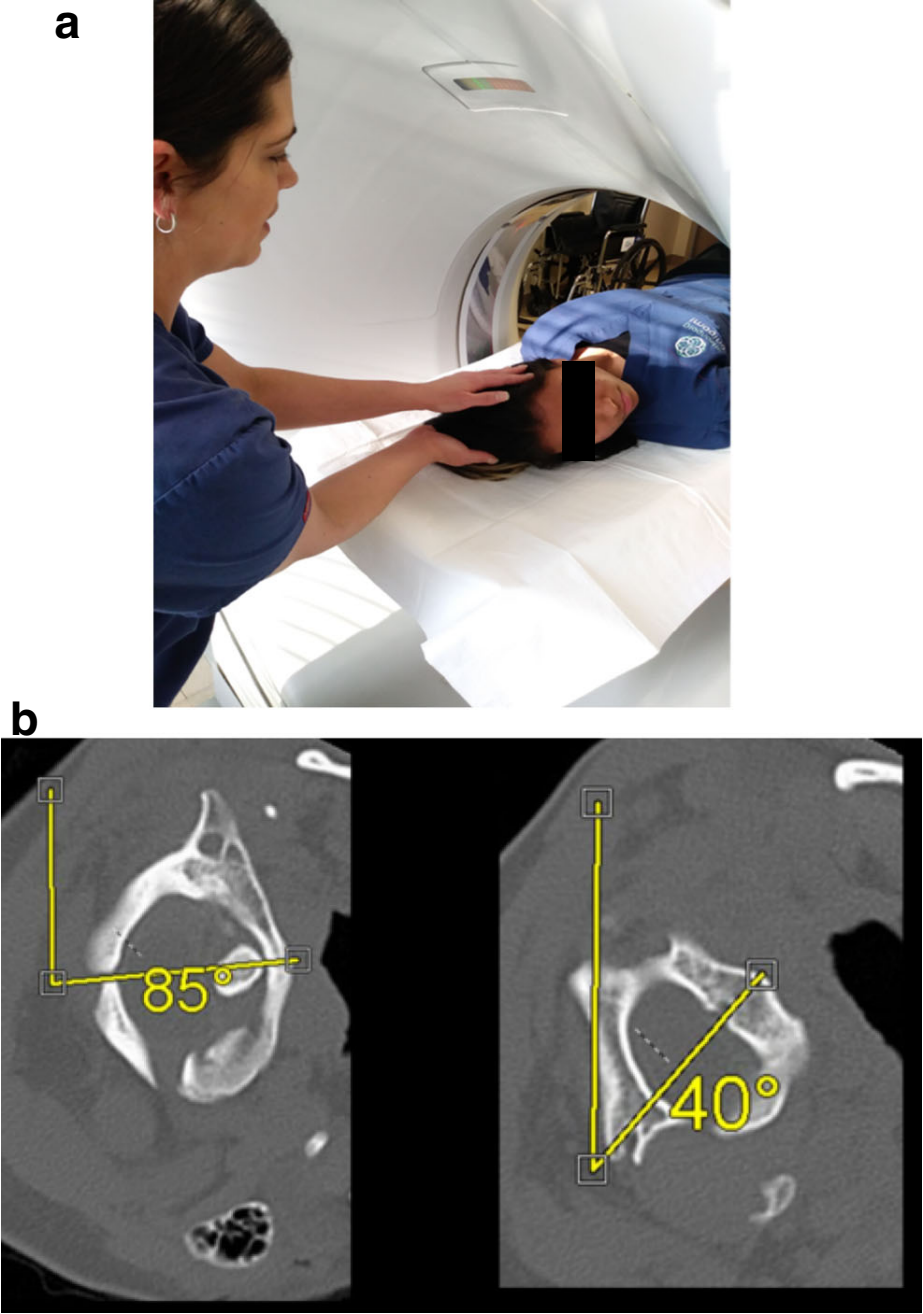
a To assess for alar ligament incompetence and possible subluxation between C1 and C2, a dynamic CT of the upper cervical spine is performed. A CT scan is performed with the patient lying supine, with the head rotated fully to the left, then rescanned with the head rotated fully to the right, as shown in b. b Axial CT view through C1 and C2, showing the angle deviation from the neutral position (12 o’clock position). The angle subtended by C1 minus the angle subtended by C2 is the angle between C1 and C2. In this case, the angle subtended between C1and C2 is 85° minus 40° equals 45°, which exceeds the pathological threshold of 42°
